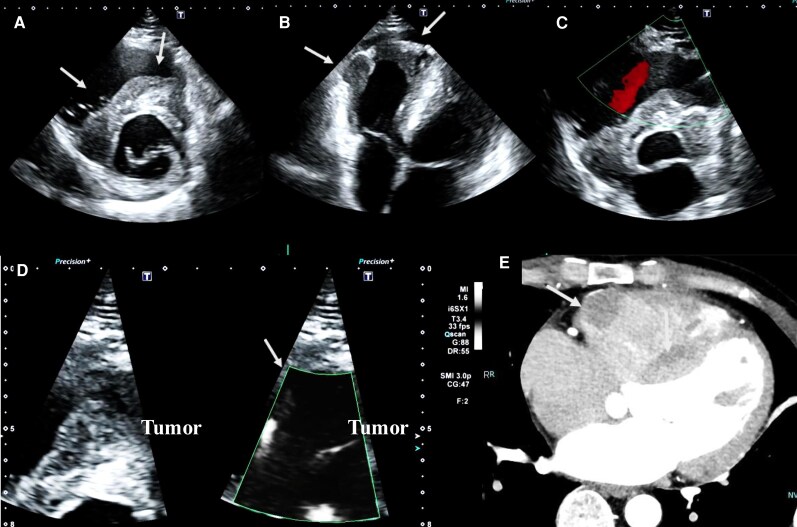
A case of malignant lymphoma. Transthoracic echocardiography scans in (*A*) a short-axis view and (*B*) a modified apical four-chamber view of the right ventricle showing intracardiac tumours with irregular margins in the right ventricular outflow tract and free wall of the right ventricle (white arrows). (*C*) Conventional colour Doppler and (*D*) superb microvascular imaging failed to detect any intratumoural blood flow signals (white arrows). (*E*) Contrast-enhanced cardiac computed tomography scan revealed lower contrast effects in the tumour (white arrows).
